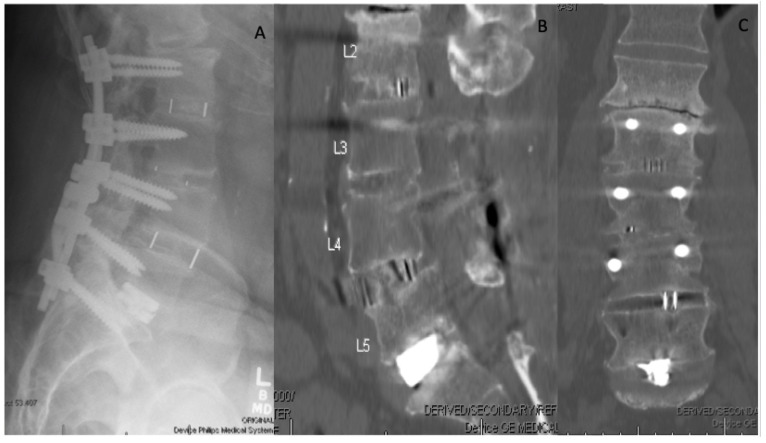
Lateral X-ray of the lumbar spine. (A) Demonstrating L2–S1 anterior interbody fusion using a combination of PEEK and titanium cages that is supported posteriorly with pedicle screw fixation. One year post-op CT sagittal (B) and coronal slices (C) demonstrate bridging callus between the interbody spaces showing successful fusion with osseointegration.
